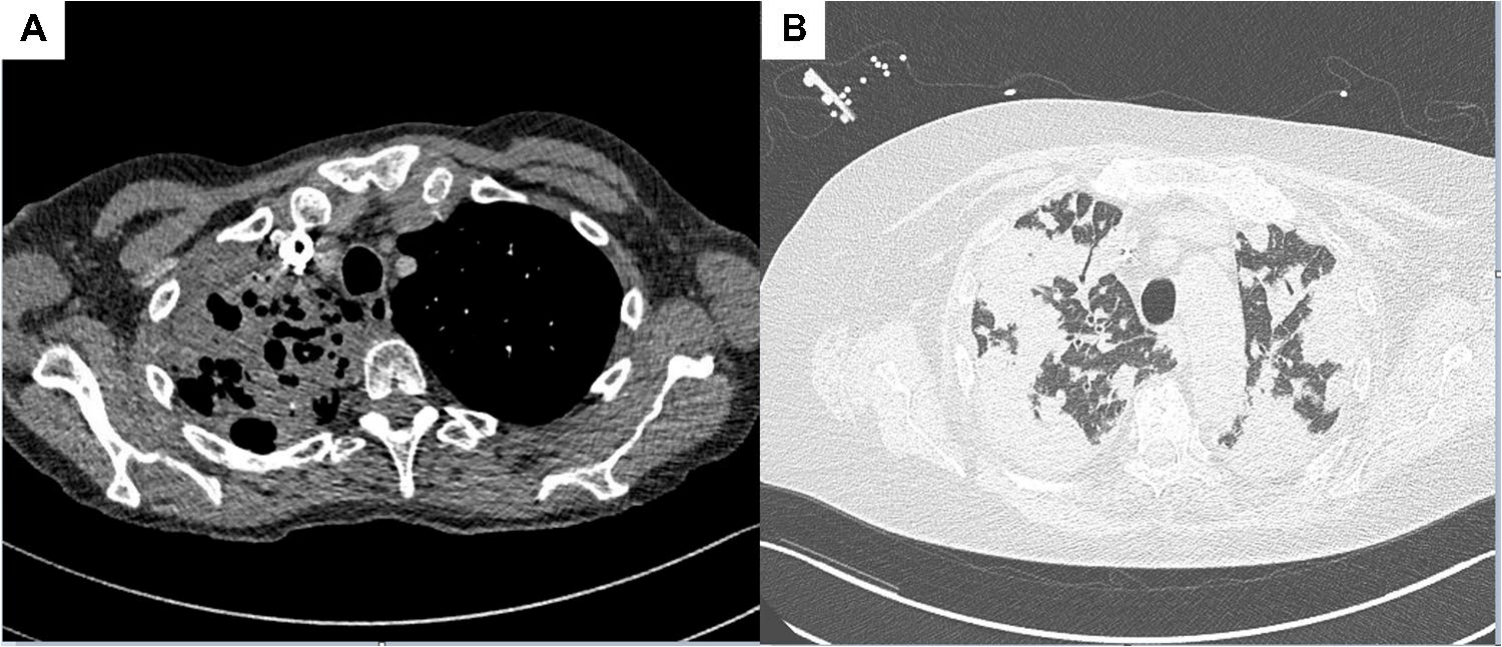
Computed tomography scans of A the first case showing consolidations in the right lung and a lung abscess in the right upper lung lobe and B the second case showing diffuse consolidations over all the lung fields
